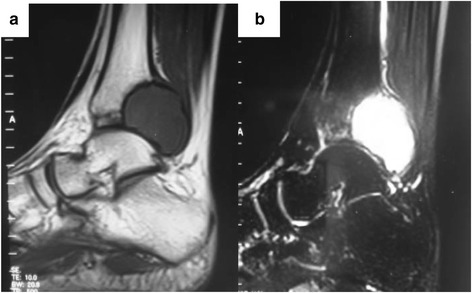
Pre-operative MRI shows that T1 low signal and T2 high signal of the lesion located in the distal of the right tibia protruded into the soft tissue. a T1 MRI. b T2 MR
